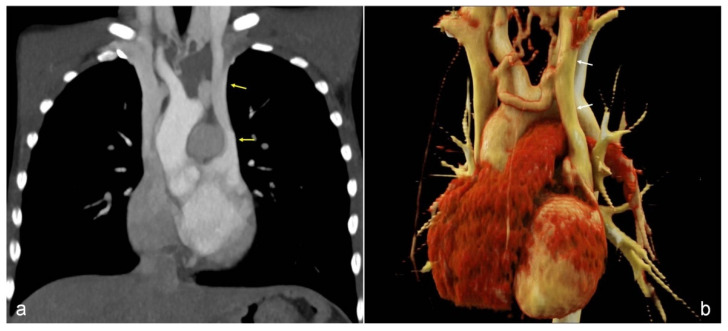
(a). Computed tomography reveals a persistent left SVC (arrows) with drainage in the left atrium and resulting right-to-left shunt; right SVC is regularly present, draining to the right atrium.(b). A3D-volume rendering image of the computed tomography confirms the condition; it allows to better appreciate a bridging vein between left and right SVS, with mean diameter of 7 mm.
